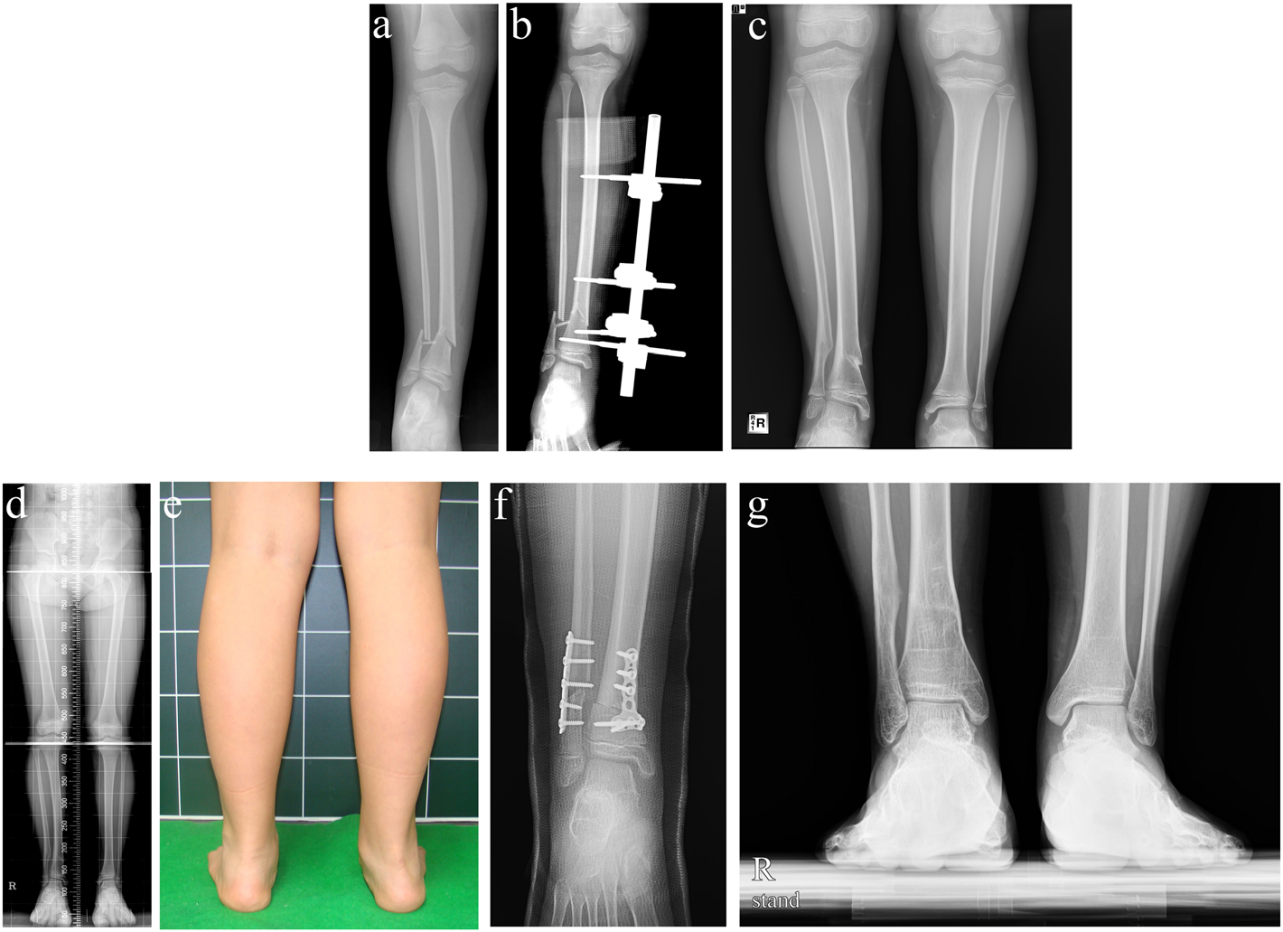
Serials radiographs of a seven-year-old girl who sustained a left distal tibiofibular fracture. (a) Anteroposterior radiograph at trauma. (b) Immediate postoperative radiograph. Treatment involved external fixation of the tibia without fibular fixation. (c) Standing anteroposterior radiographs after 1 year shows 20° valgus angulation. (d) Standing anteroposterior radiograph after 3 years of follow-up shows 15° valgus angulation and a 15-mm tibial overgrowth. (e) Gross image of valgus angulation around the ankle joint. (f) A postoperative radiograph 3 years after corrective osteotomy and shortening. (g) A radiograph 3 years after corrective osteotomy shows an excellent correction.
